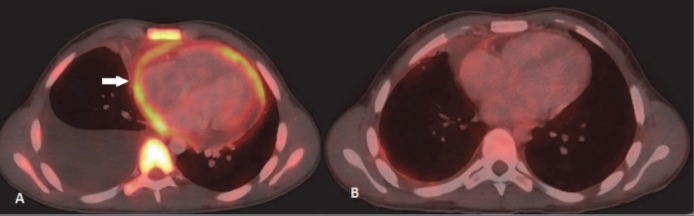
NHL with pericardial involvement. (A) FDG avid pericardial involvement is seen. Associated marrow involvement and pleural effusion is also seen. (B) Post 3 cycle chemotherapy shows complete metabolic response
